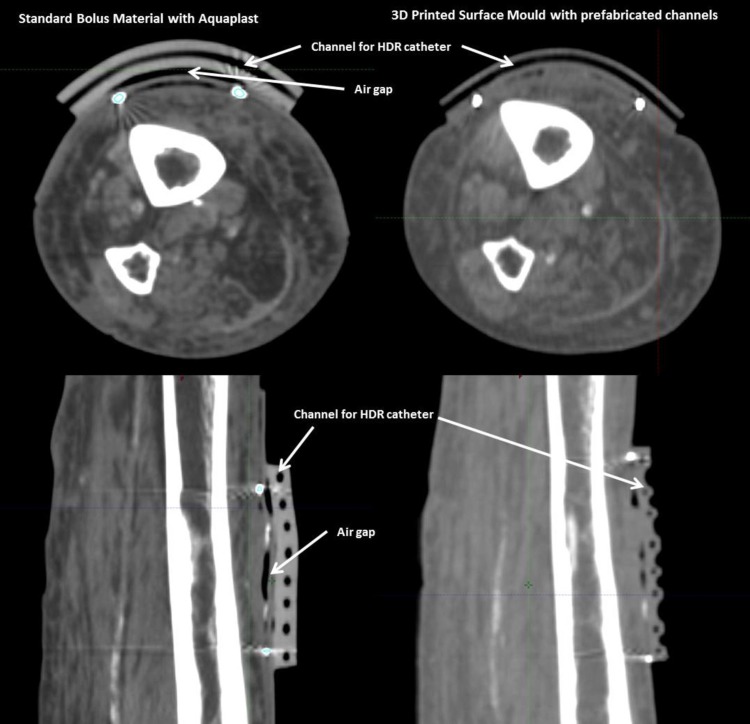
Axial and sagittal computed tomography images with standard vs. 3D printed bolus
